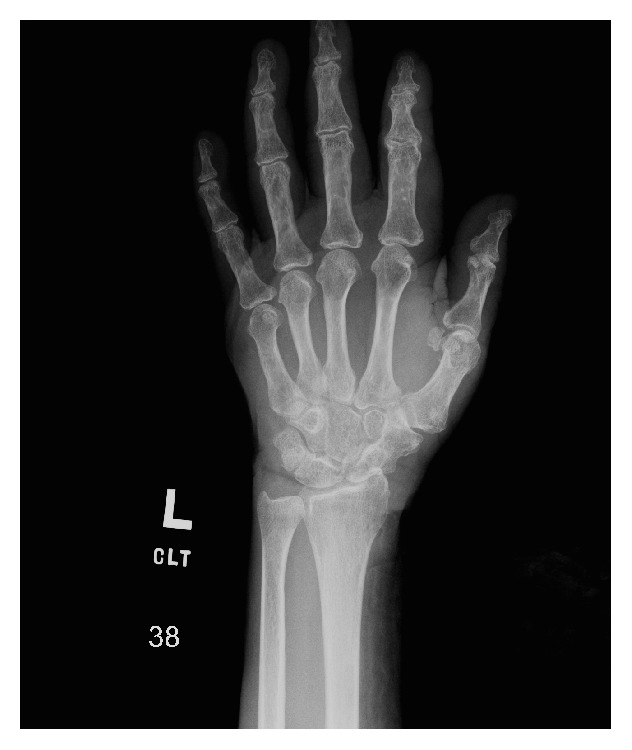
Frontal radiograph of patient 2 demonstrates isolated osseous scaphotrapezial coalition.
